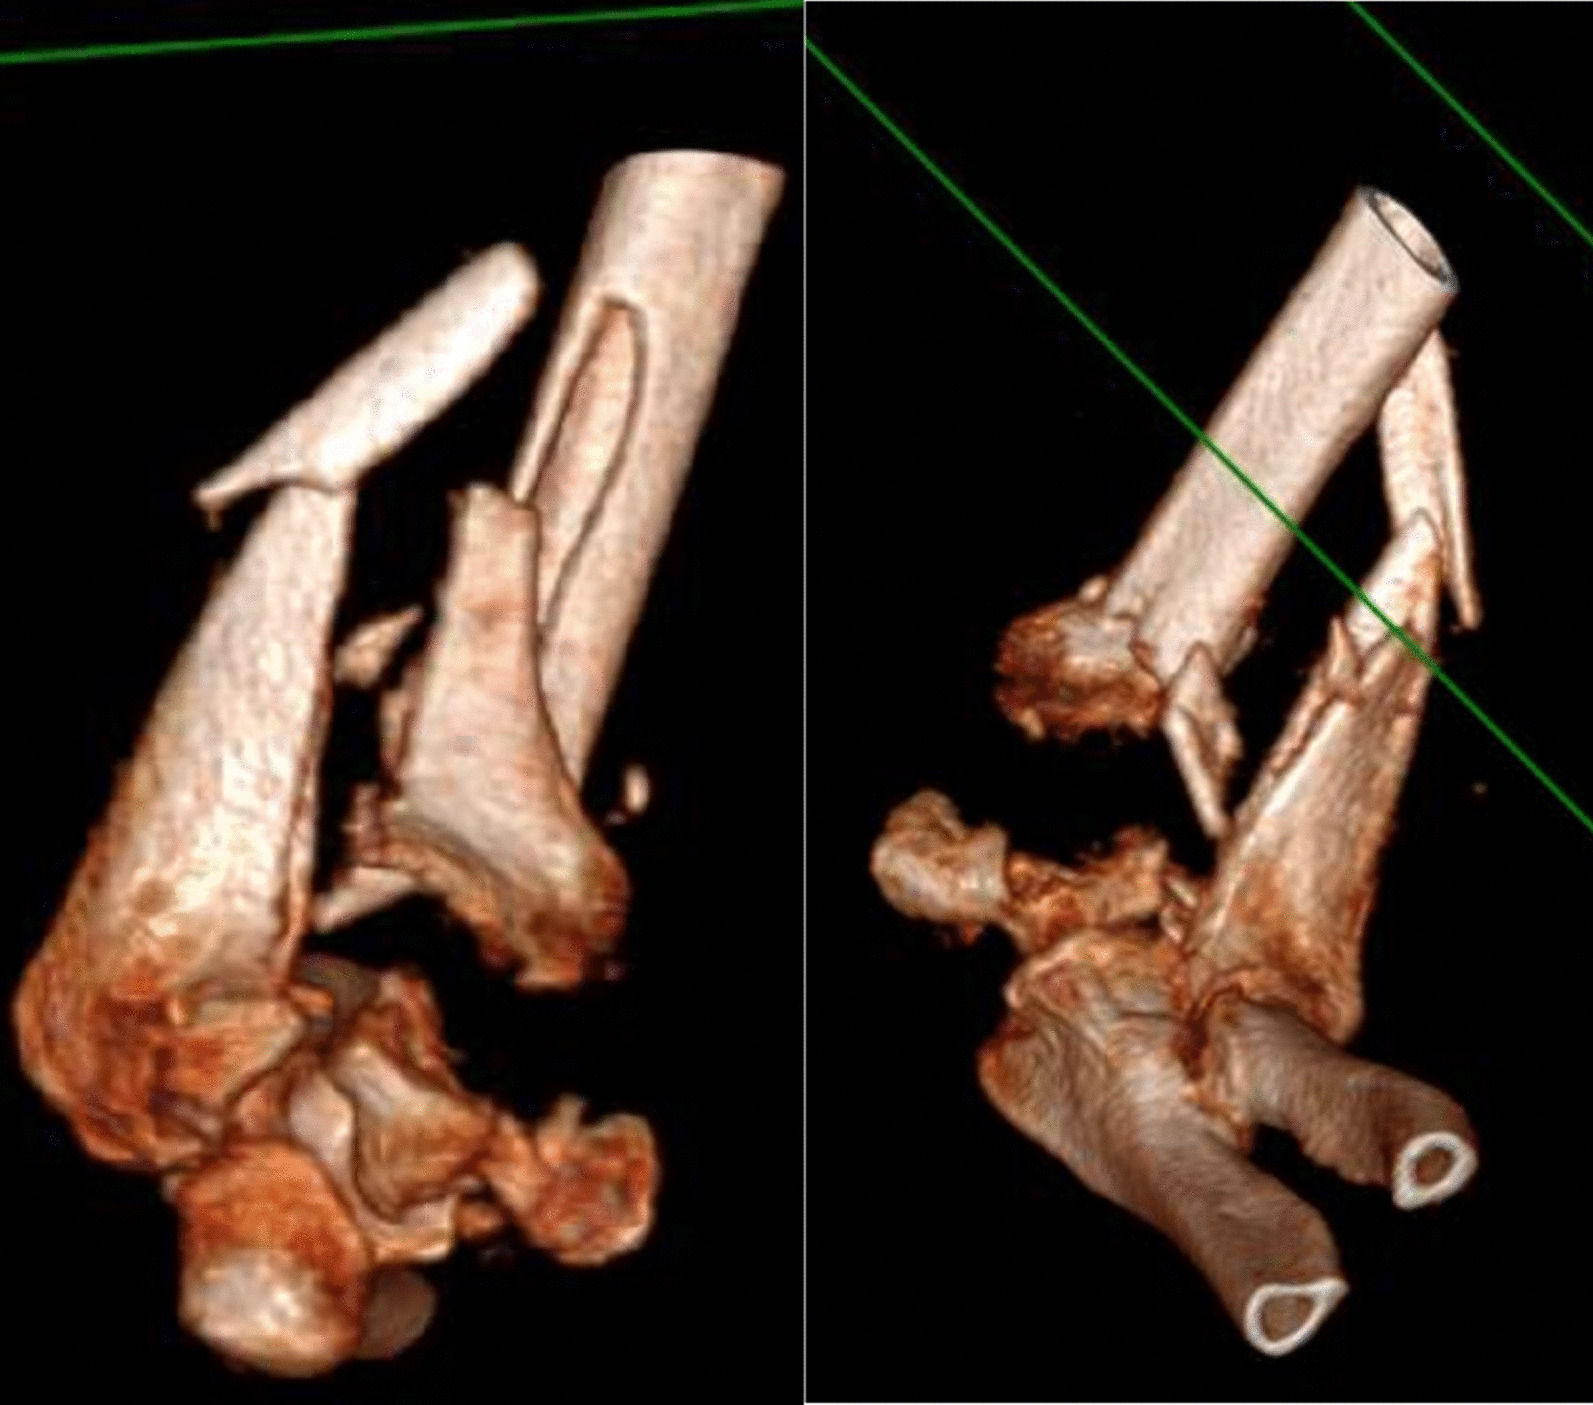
Preoperative CT scan, 3D bone reconstructions
